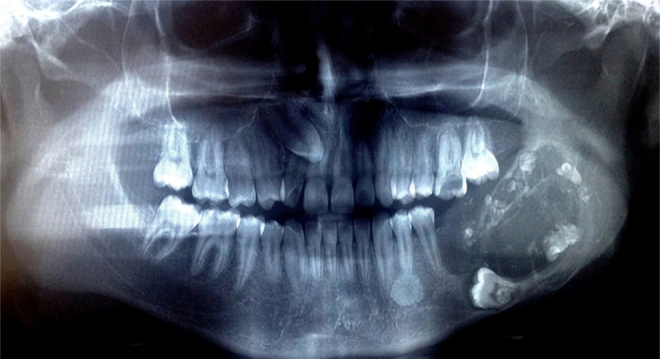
Panoramic radiograph showing multilocular extensive radiolucent lesion with radiopaque foci in the left mandible, associated with impacted molar (36).
